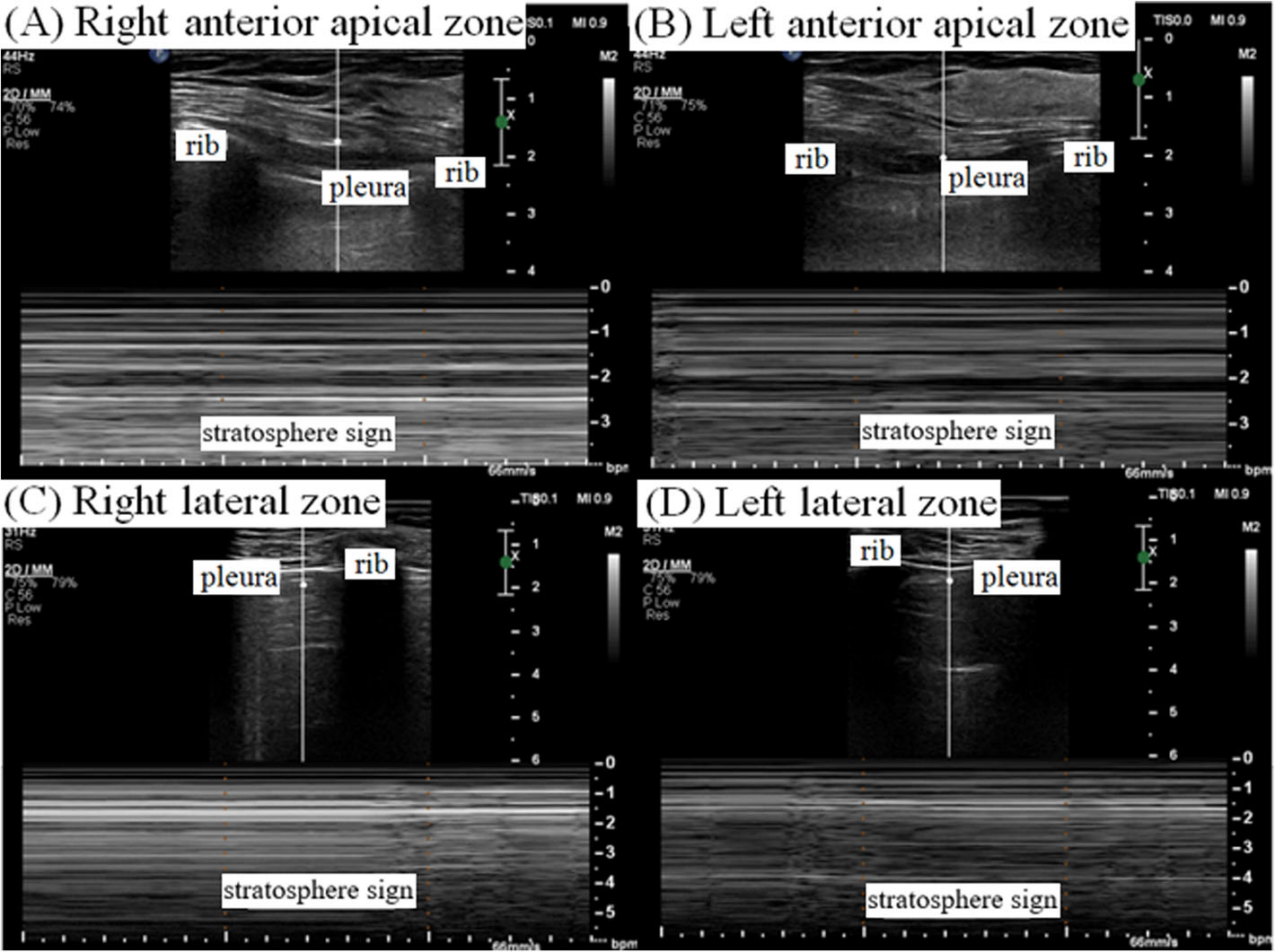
Stratosphere sign on point-of-care lung ultrasound. a Right anterior apical zone. b Left anterior apical zone. c Right lateral zone. d Left lateral zone
